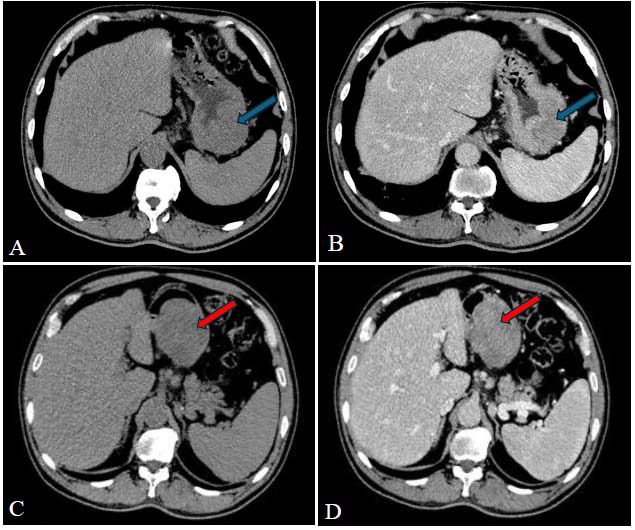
CT cross-section of the lesion. The blue arrows in (A) and (B) show the CT plain scan and enhanced images of gastric body-gastric fundus lesions, respectively. The density of the plain scan lesions is slightly lower than the muscle density, and the enhanced scan is moderately enhanced. The red arrows in (C) and (D) show the CT plain scan and enhanced images of the gastric angle lesions, respectively. The enhancement shows moderate enhancement, the low-density area is gradually strengthened, and the enhancement gradually becomes uniform.
